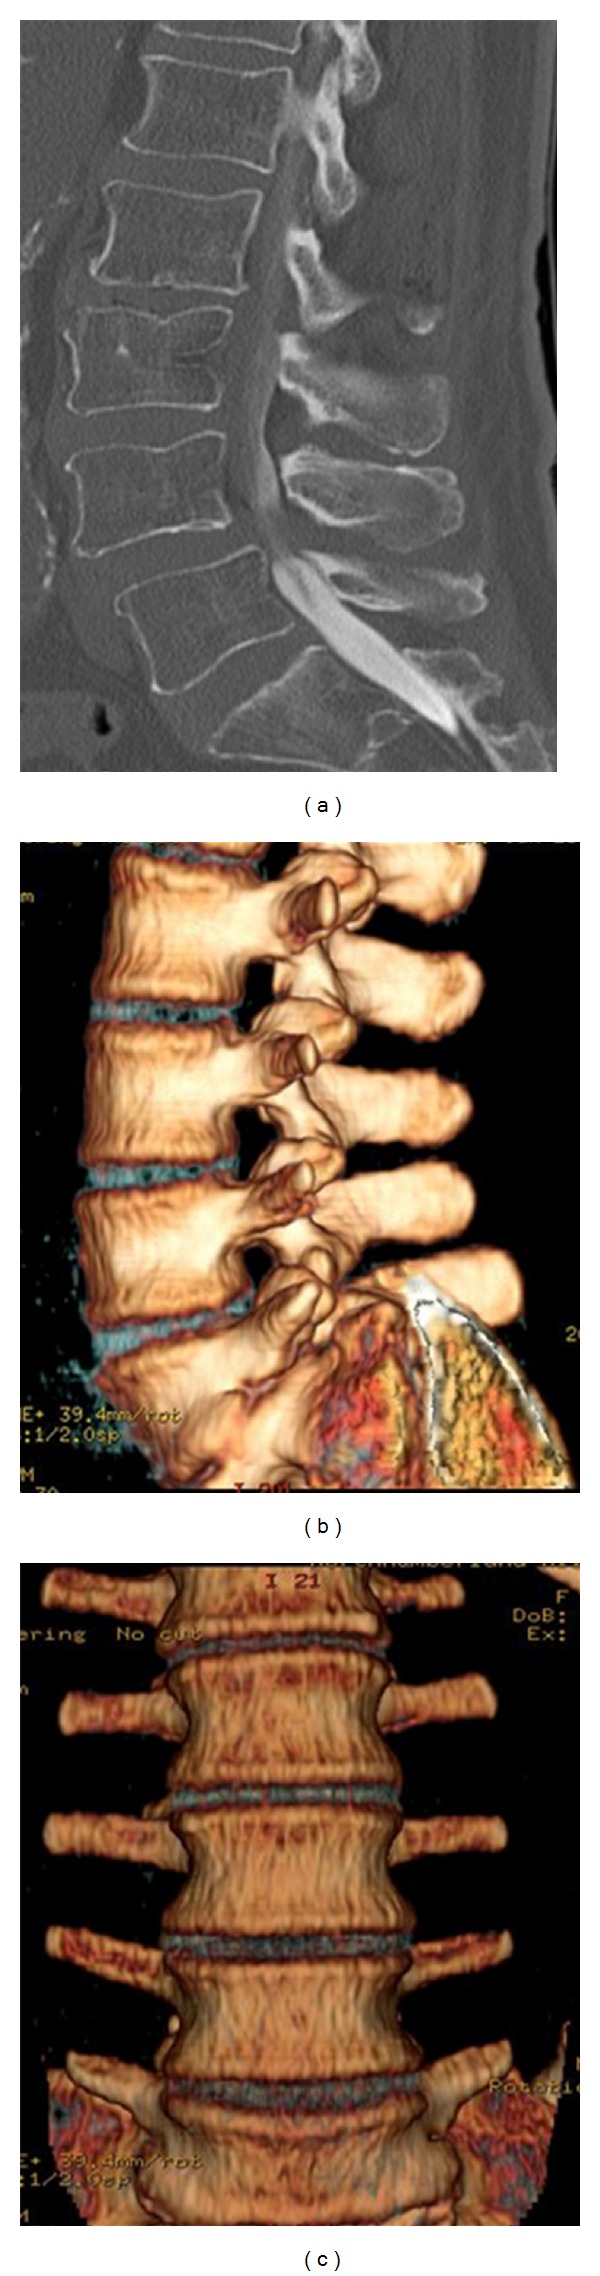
CT scans. (a) Grade I spondylolisthesis. ((b) and (c)) 3D reconstruction, lateral and anterior views.
